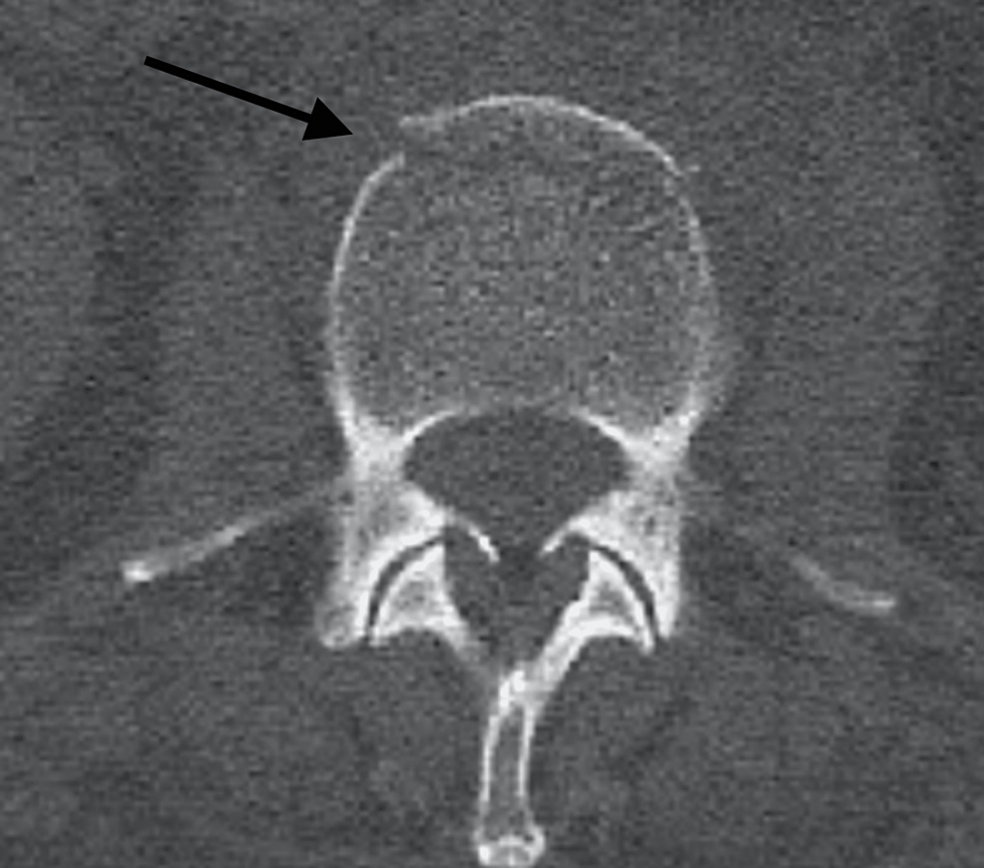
CT scan with axial view showing a nondisplaced L2 vertebral body fracture
